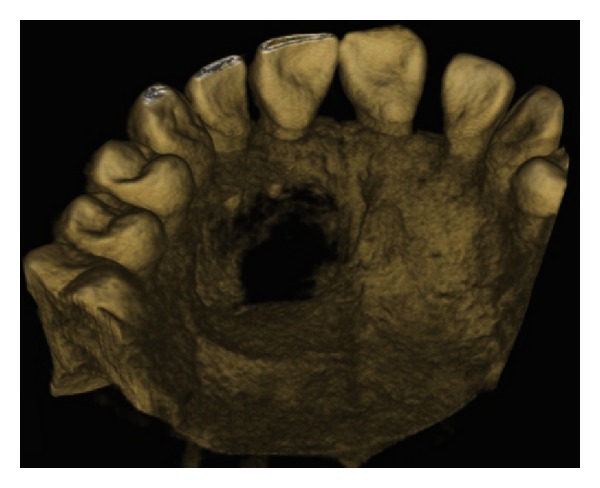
Reconstructed 3D CBCT image.
